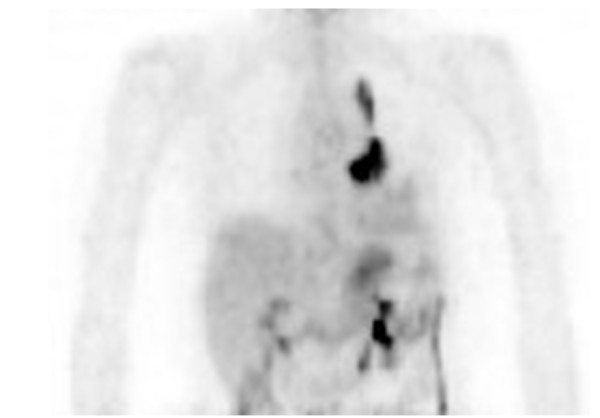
Positron emission tomography (PET) imaging showing the uptake of FDG at the lower and upper lesions in the left lung.
